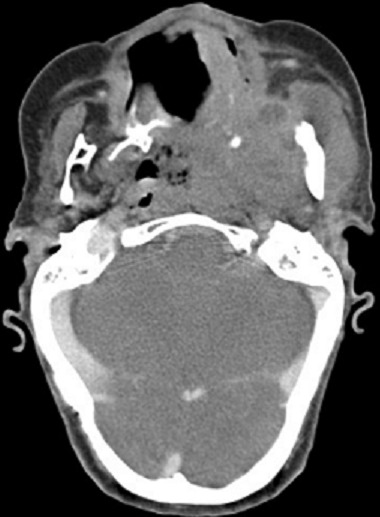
Axial post-contrast CT demonstrates tissue thickening of the hard palate extended to the masseter muscle and the nasopharynx
